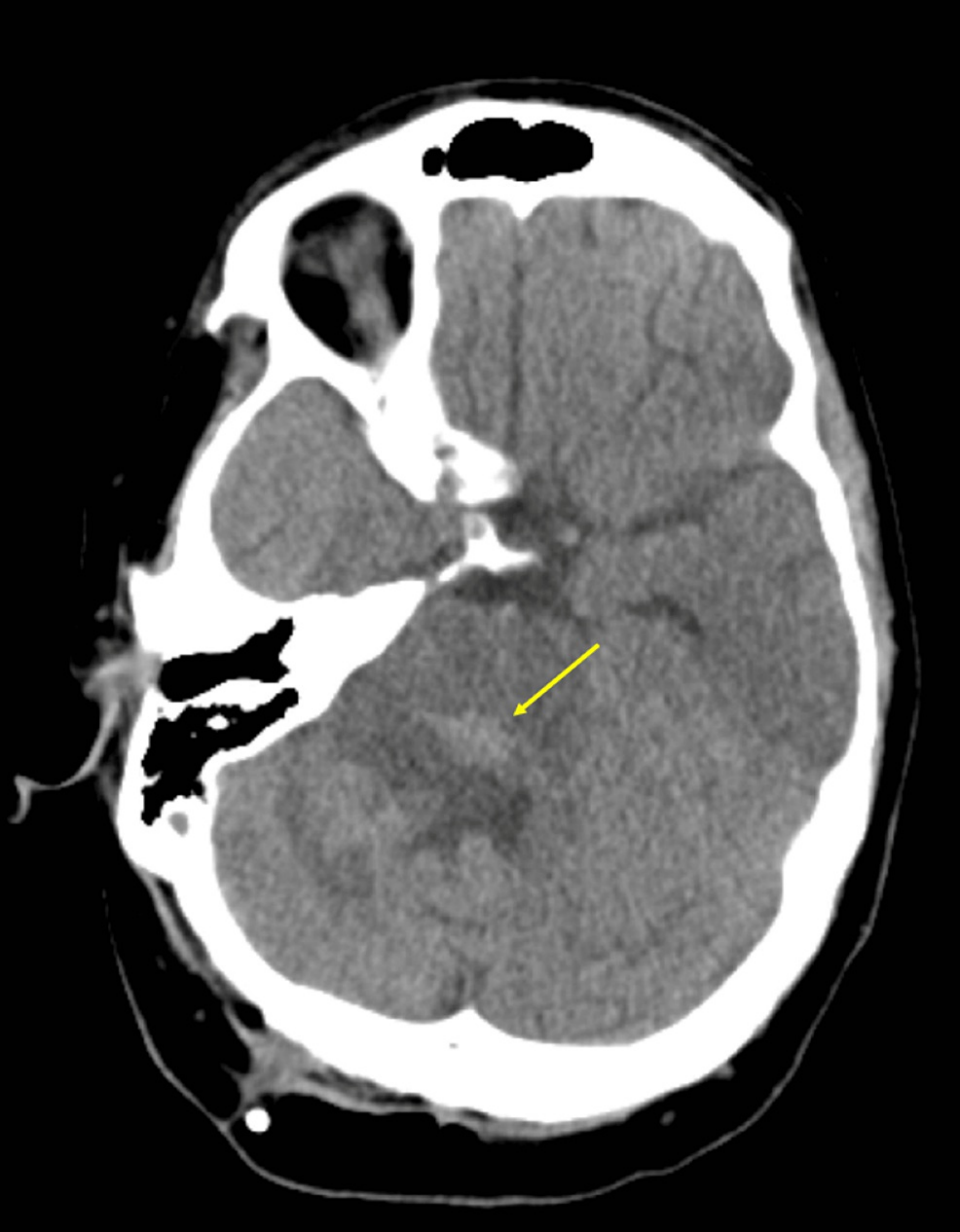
Axial non-contrast CT of the brain analyzed by the attending radiologist. The image shows post-surgical changes after a right parietal ventriculostomy catheter placement with mildly dilated ventricles. There is a focus on ill-defined hyperattenuation noted in the posterior pons (seen by the arrow), which corresponds to an area of hypercellularity seen on the follow-up MRI (area of diffusion restriction).
